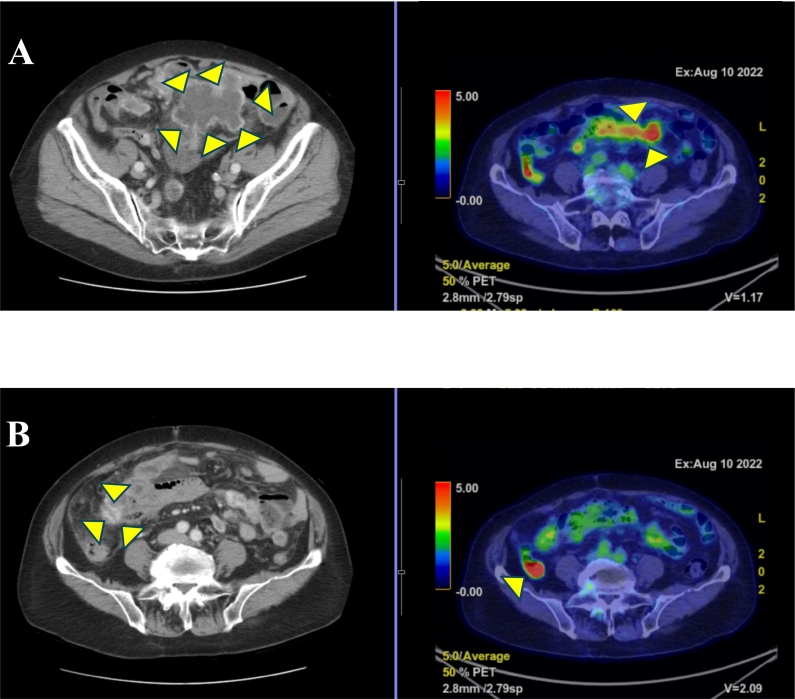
Abdominal CT and PET-CT findings. A and B: CT showed fluid accumulation with contrast effect in the pelvis and abdominal cavity. PET-CT showed significant FDG accumulation. CT, computed tomography; FDG, fluorodeoxyglucose; PET, positron emission tomography.
